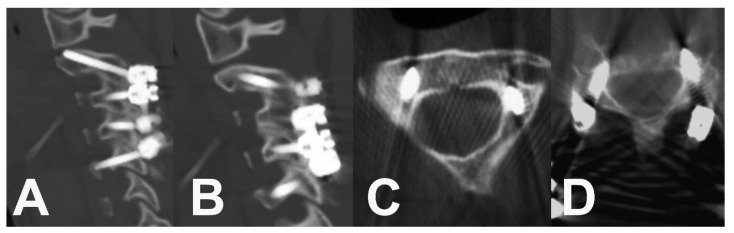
CT scan shows the fusion one year after surgery. (A,B) Sagittal slices of the fused cervical spine from C2 to C5. (C) Axial slice of C2 with transpedicular screw insertion and a high vertebral artery position on the left side. (D) Axial slice of the C5 bilateral transpedicular fixation.
